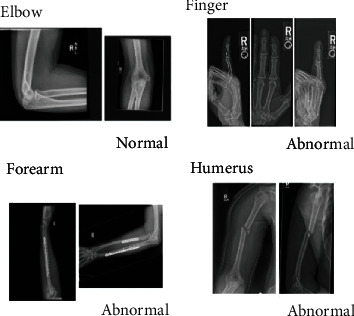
An example from the MURA dataset is Towards Radiologist-Level Abnormality Identification in Musculoskeletal Radiographs.
